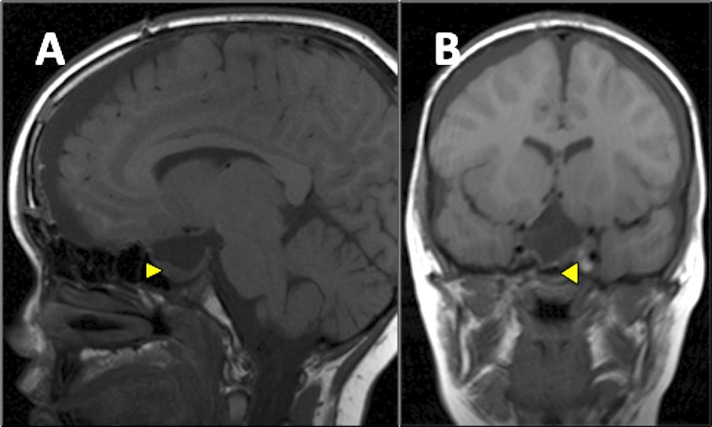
Postoperative T1-weighted MRI brain (sagittal view [panel A], coronal view [panel B]) Shows subtotal resection of the craniopharyngioma with only a small intrasellar residuum (arrowhead).
